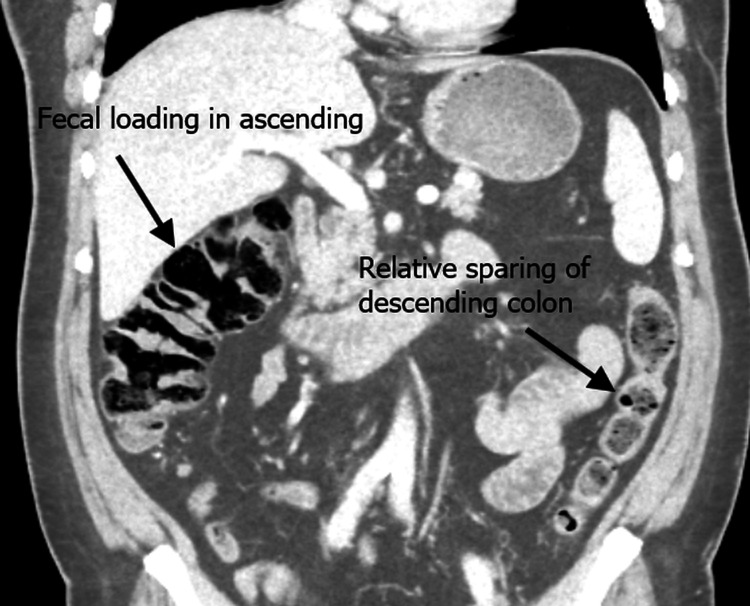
Coronal CT scan demonstrating fecal loading in the ascending colon with relative sparing of the descending colon
